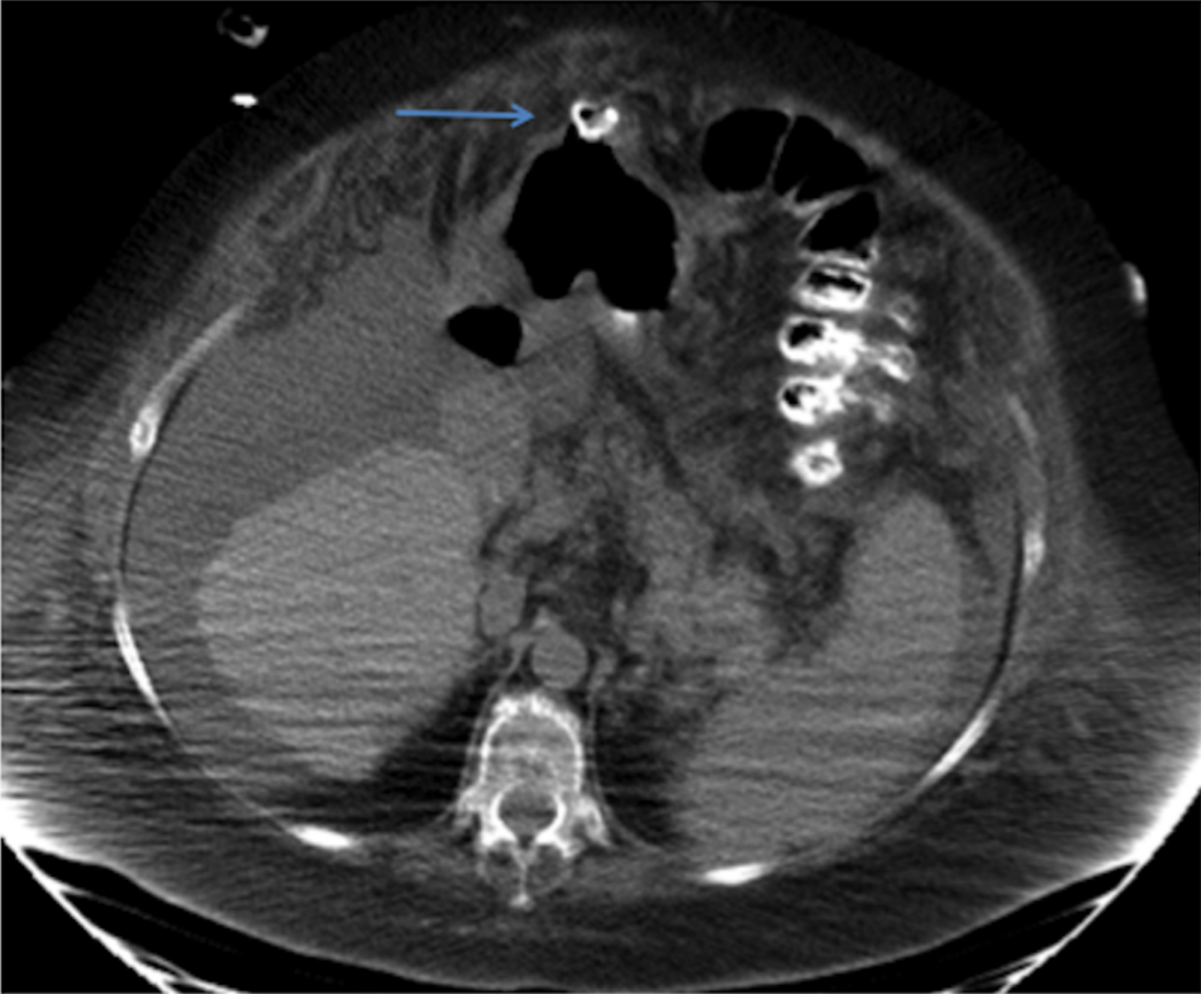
An axial view of the computed tomography scan of the abdomen demonstrating the erosion of the percutaneous endoscopic gastrostomy (PEG) tube through the stomach wall i.e. "buried bumper"
